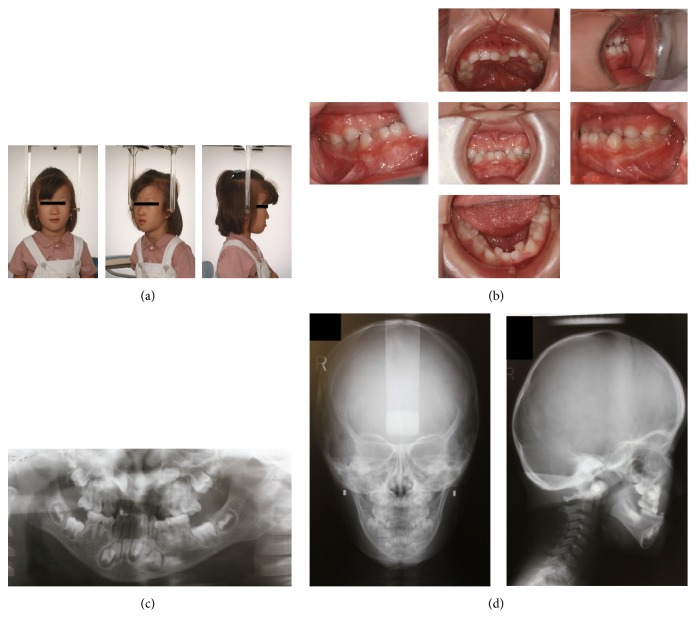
Pretreatment photographs (5 years and 1 month). (a) Extraoral photographs. (b) Intraoral photographs. (c) Orthopantomograms. (d) Posteroanterior and lateral cephalograms.
